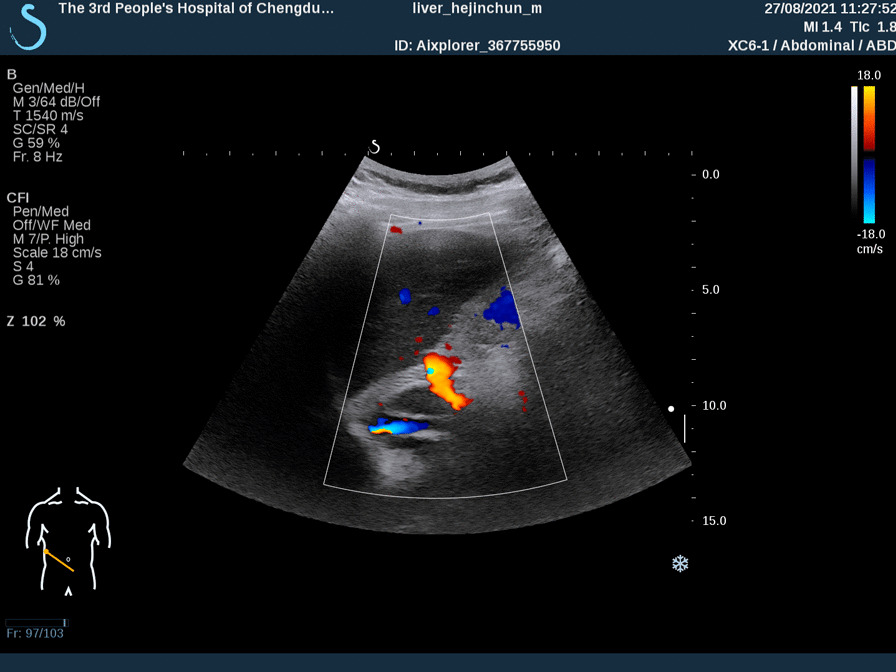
Liver ultrasound shows tubular hyperechoic shadow between the right hepatic vein and the right branch of the portal vein without blood flow signal in it
